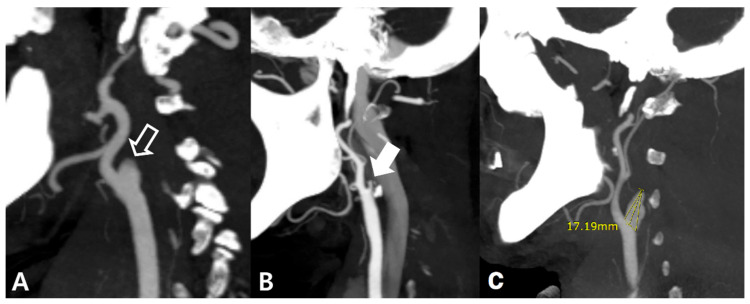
Images of the proximal internal carotid artery occlusion on computed tomography angiography ((A): blunt shape, empty arrow; (B): not-blunt shape, white arrow; (C): length of the ICA stump measurement).
